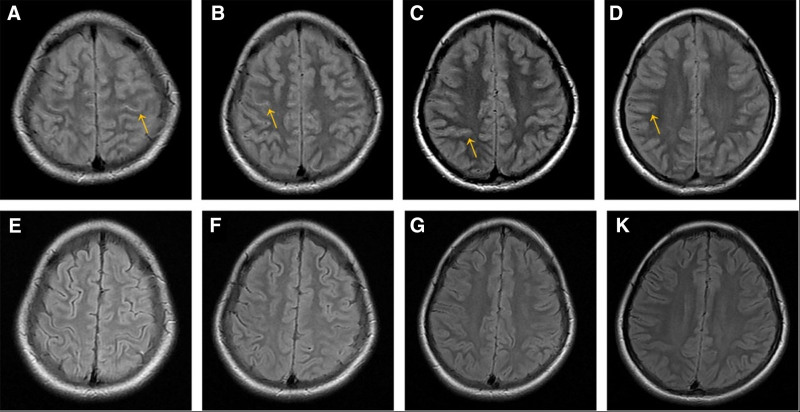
Magnetic resonance imaging findings. First day of admission, brain MRI T2-weighted fluid attenuated inversion recovery sequences revealed prominent linear signals (A–D). However, at discharge, 50th day of hospitalization, the majority of the linear signals seen in head MRI T2 sequences were resolved (E–K). MRI = magnetic resonance imaging.
